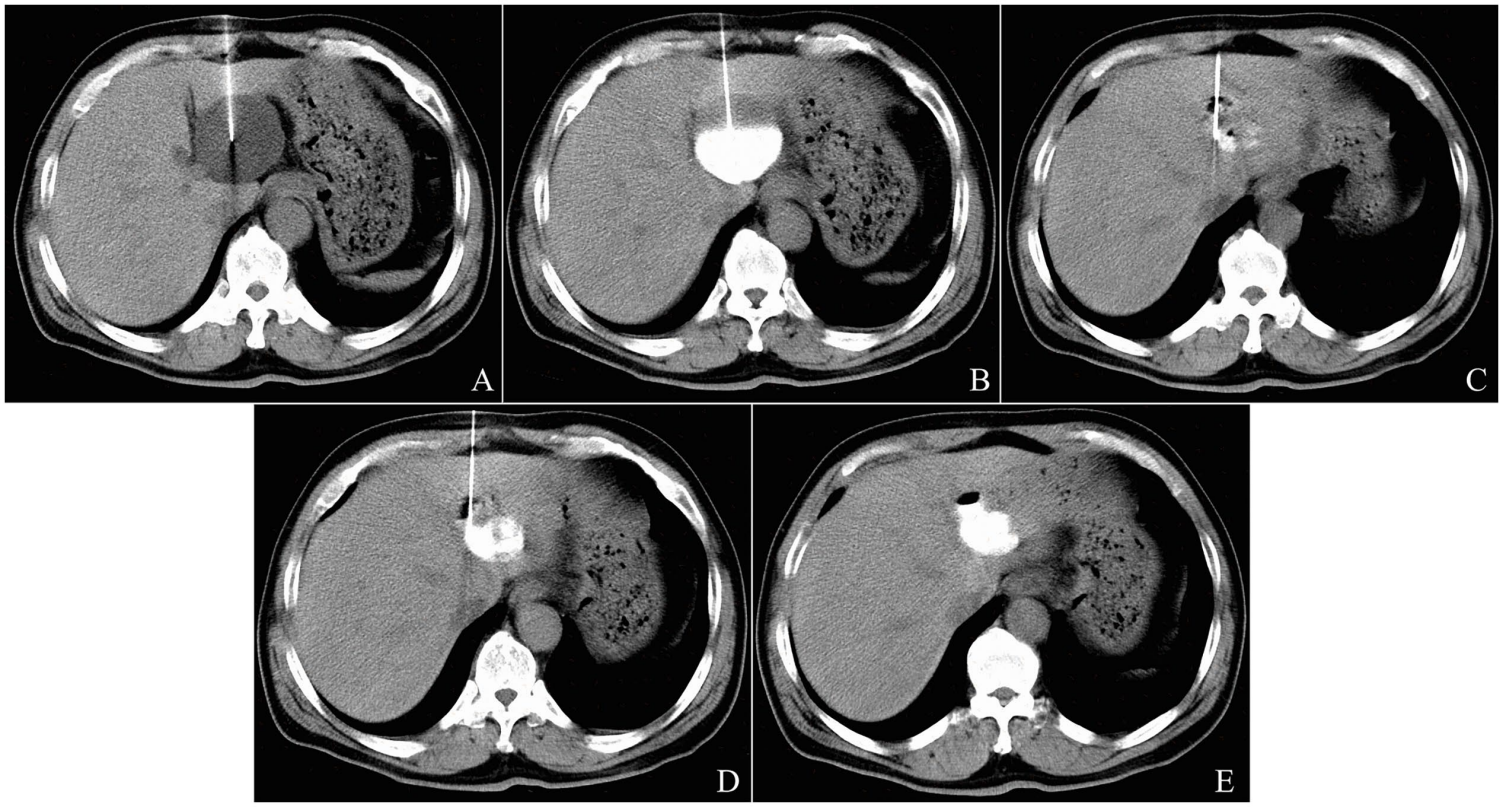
The single-pass single-needle puncturing technique of CT-guided percutaneous needle aspiration and bleomycin sclerotherapy for a 67-year-old male patient with solitary hepatic cyst. A 10-cm 21-gage Chiba needle was inserted into the cyst in the left liver lobe under CT guidance (A). After the first 10 mL of aspirated cyst fluid was sent to the laboratory for examination, 10 mL of diluted contrast media was injected into the cyst to confirm that there was no communication between the cyst and the biliary system (B). The cyst fluid was then evacuated almost completely (C). The repeat CT scan showed no leakage after injection of the half-dose Bleomycin solution contained diluted contrast media (D). The postprocedure CT scan showed that bleomycin solution was stayed in the cyst cavity (E).
